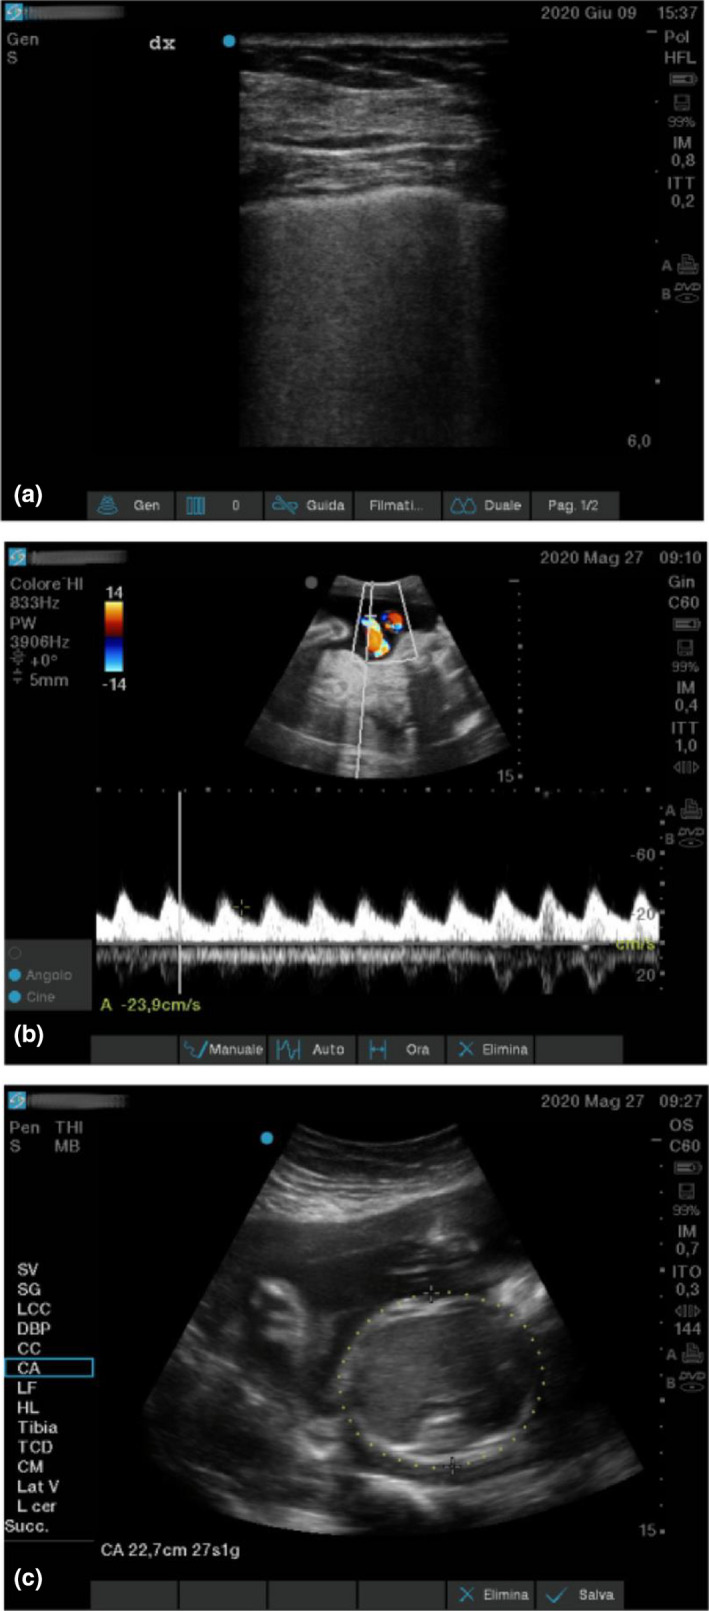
Ultrasounds. Panel A: Lung ultrasound showing multiple confluent B lines and pleural line thickening. Panel B: Fetal monitoring through umbilical artery doppler. Panel C: Fetal monitoring through biometrical measurements, for example, head circumference: showing regular fetal development
